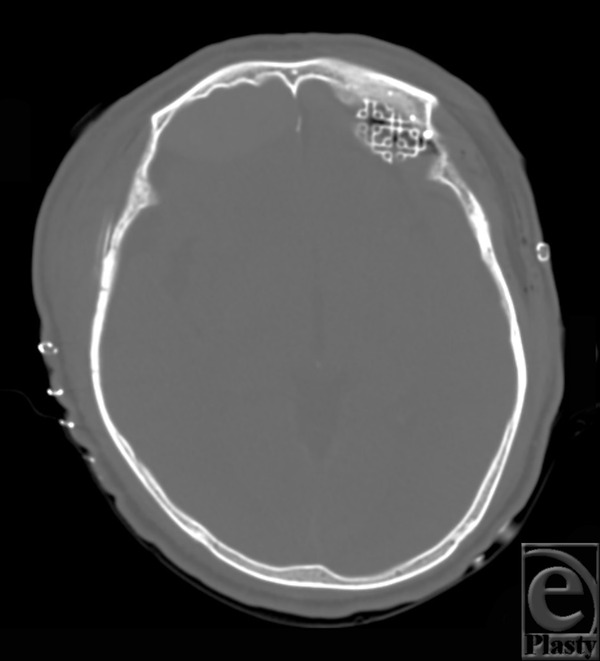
Brain computed tomography after the second operation, axial view. A displaced orbital roof fracture segment has been removed, and the defect of the orbital floor was reconstructed with a titanium mesh plate.
